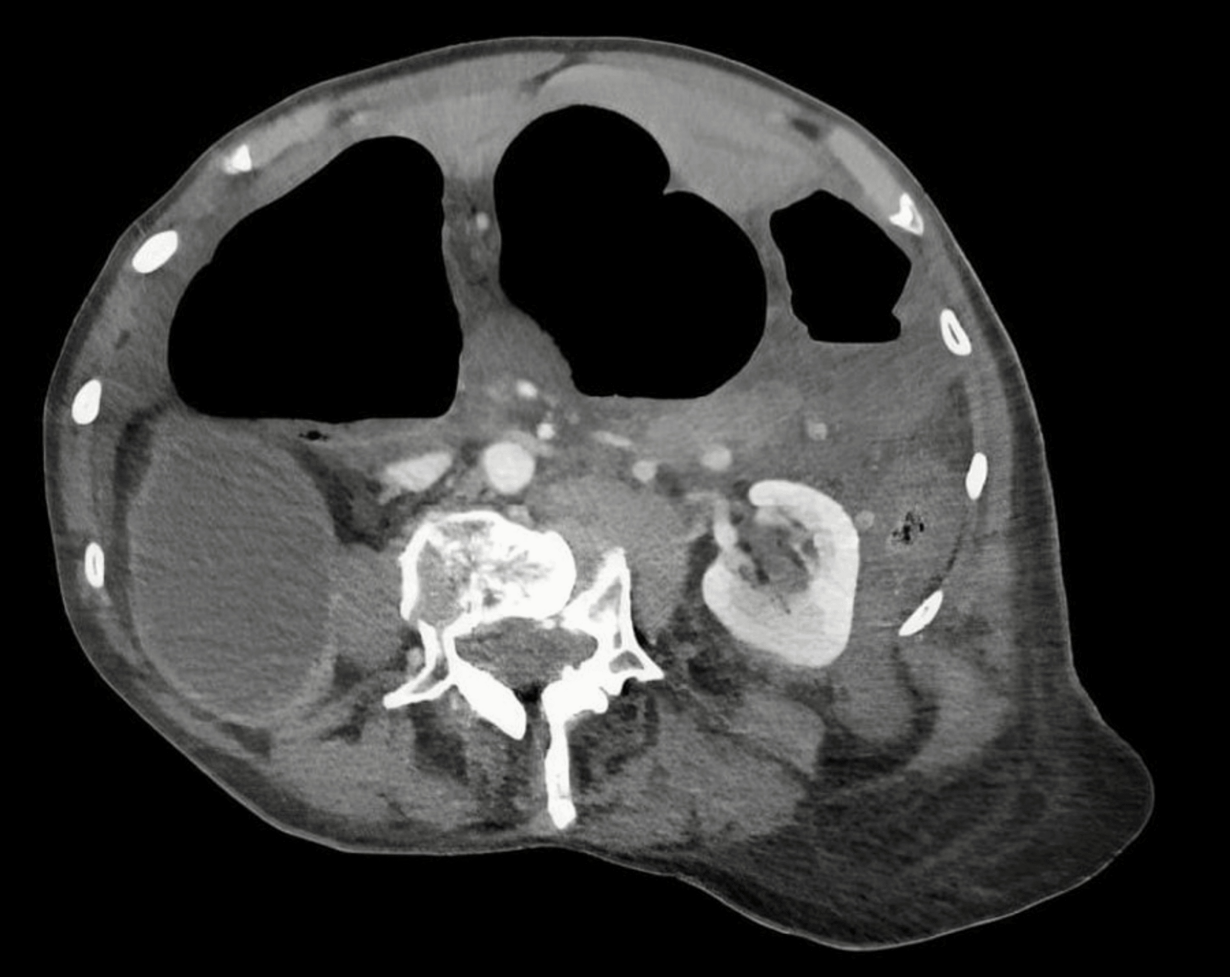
CT image suggesting volvulus
